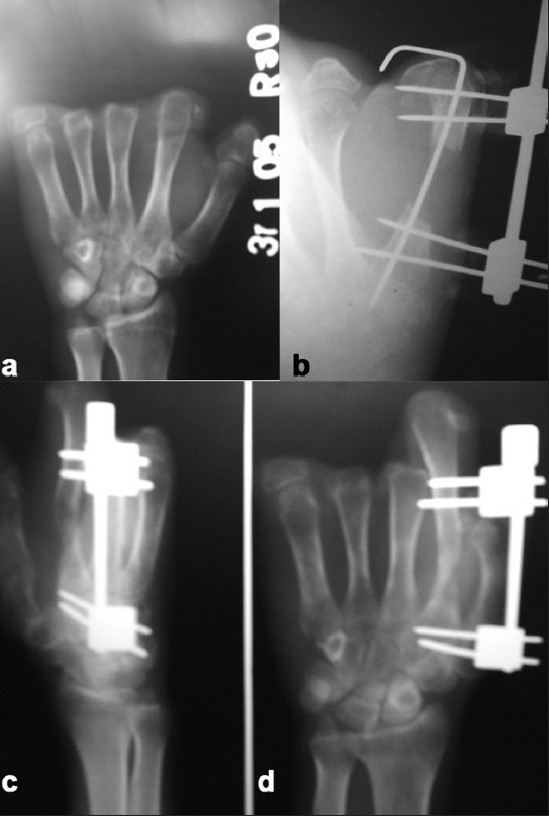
(a) X-ray (anteroposterior view) of hand showing amputated phalanges. (b) X-ray (anteroposterior view) of hand showing metacarpal lengthening in progress; K-wire and distractor *in situ*. (c, d) X-ray (oblique and anteroposterior views) of hand showing regenerate with distractor in situ
